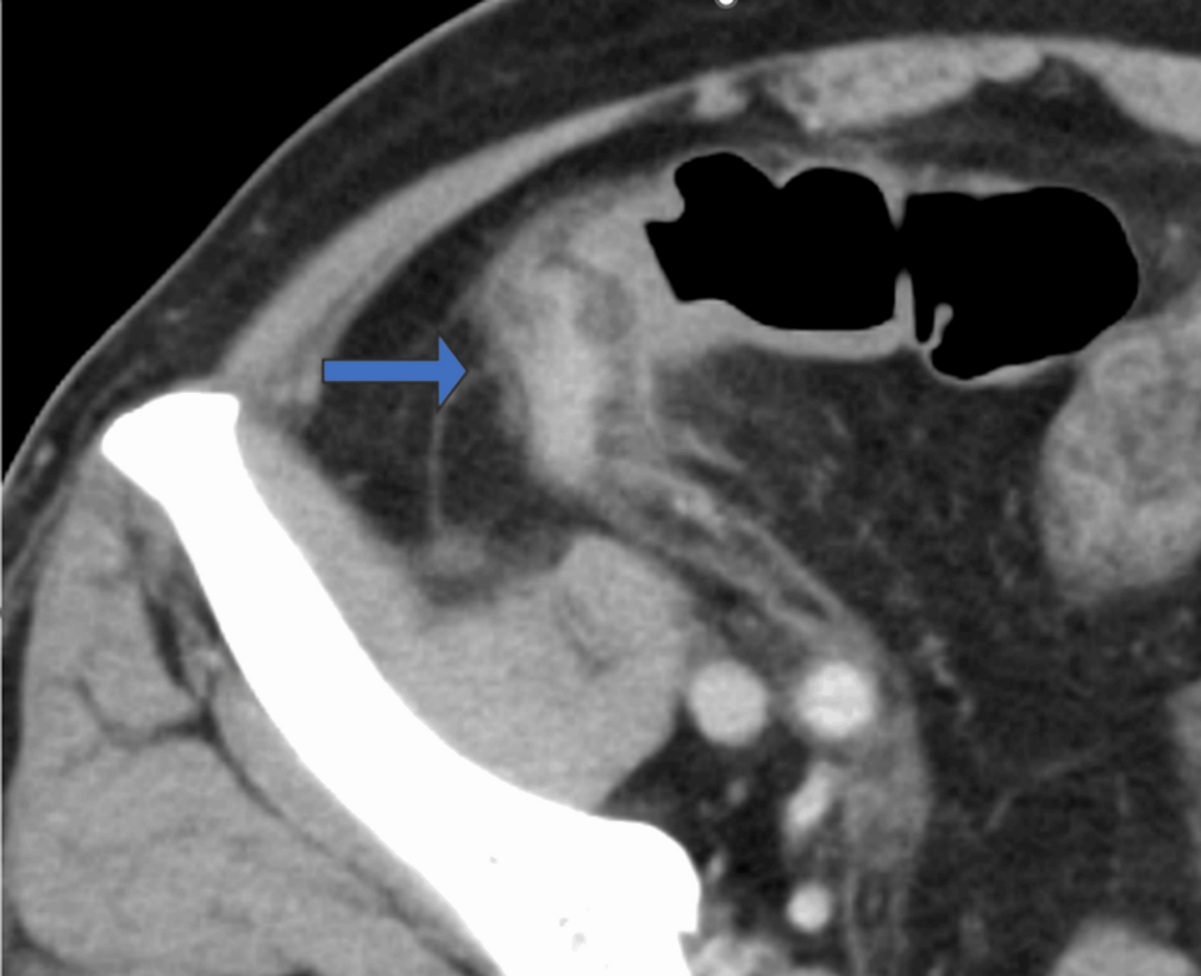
Contrast-enhanced CT revealed appendiceal wall thickening and periappendiceal fat stranding (blue arrow).
